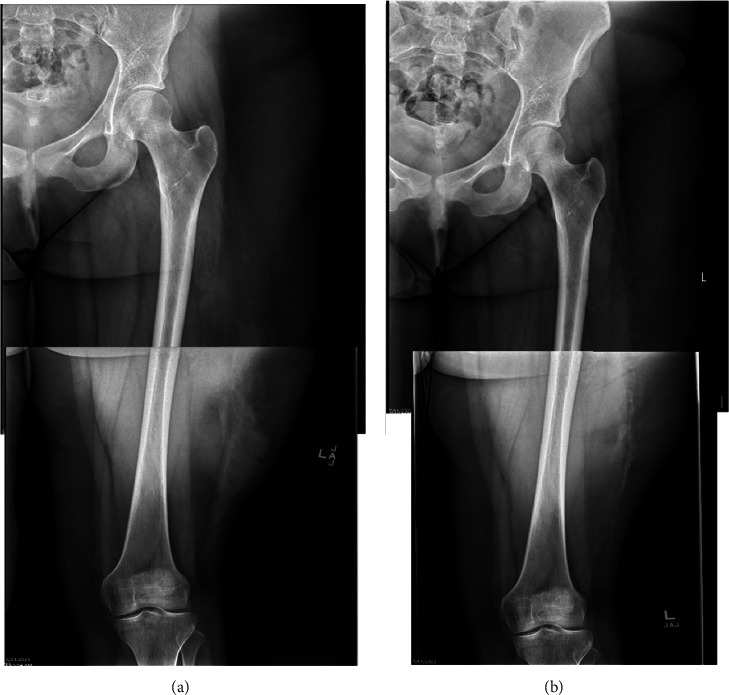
Initial AP femur radiograph of a 17-year-old female who sustained an ATV accident. Significant soft tissue swelling is noted laterally without any fracture. (a) Initial injury and (b) 13 months post-injury.
